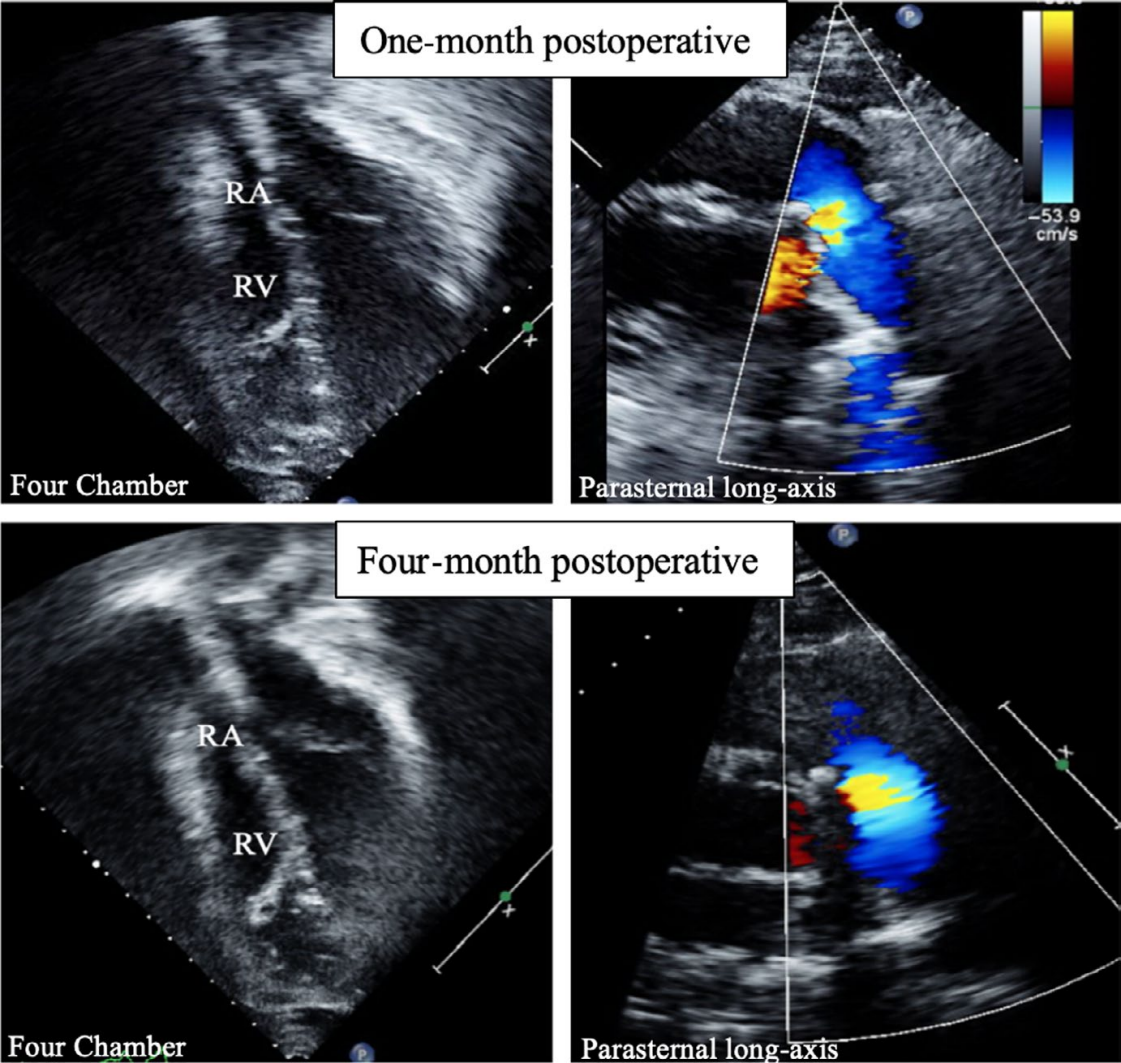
One‐ and four‐month postoperative four‐chamber and parasternal long‐axis views demonstrating normalization of RV volume and no PI, respectively
